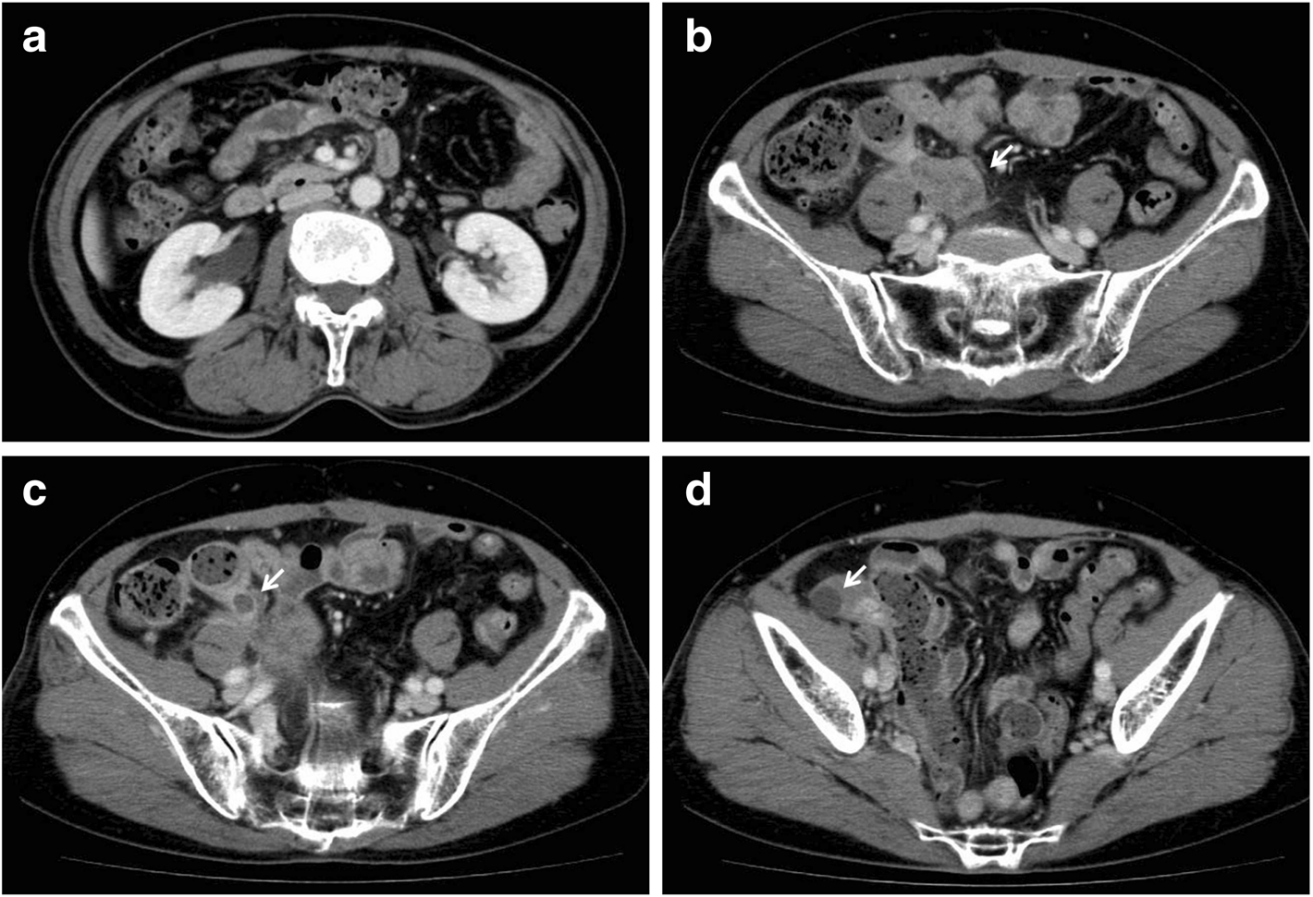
Re-examination by enhanced computed tomography (CT) scan. The CT scan shows right hydronephrosis (a), a tumorous lesion in the mesentery (b), and persistent encapsulated abscesses on the mesenteric side (c) and on the outside (d) of the ileocecal region.
